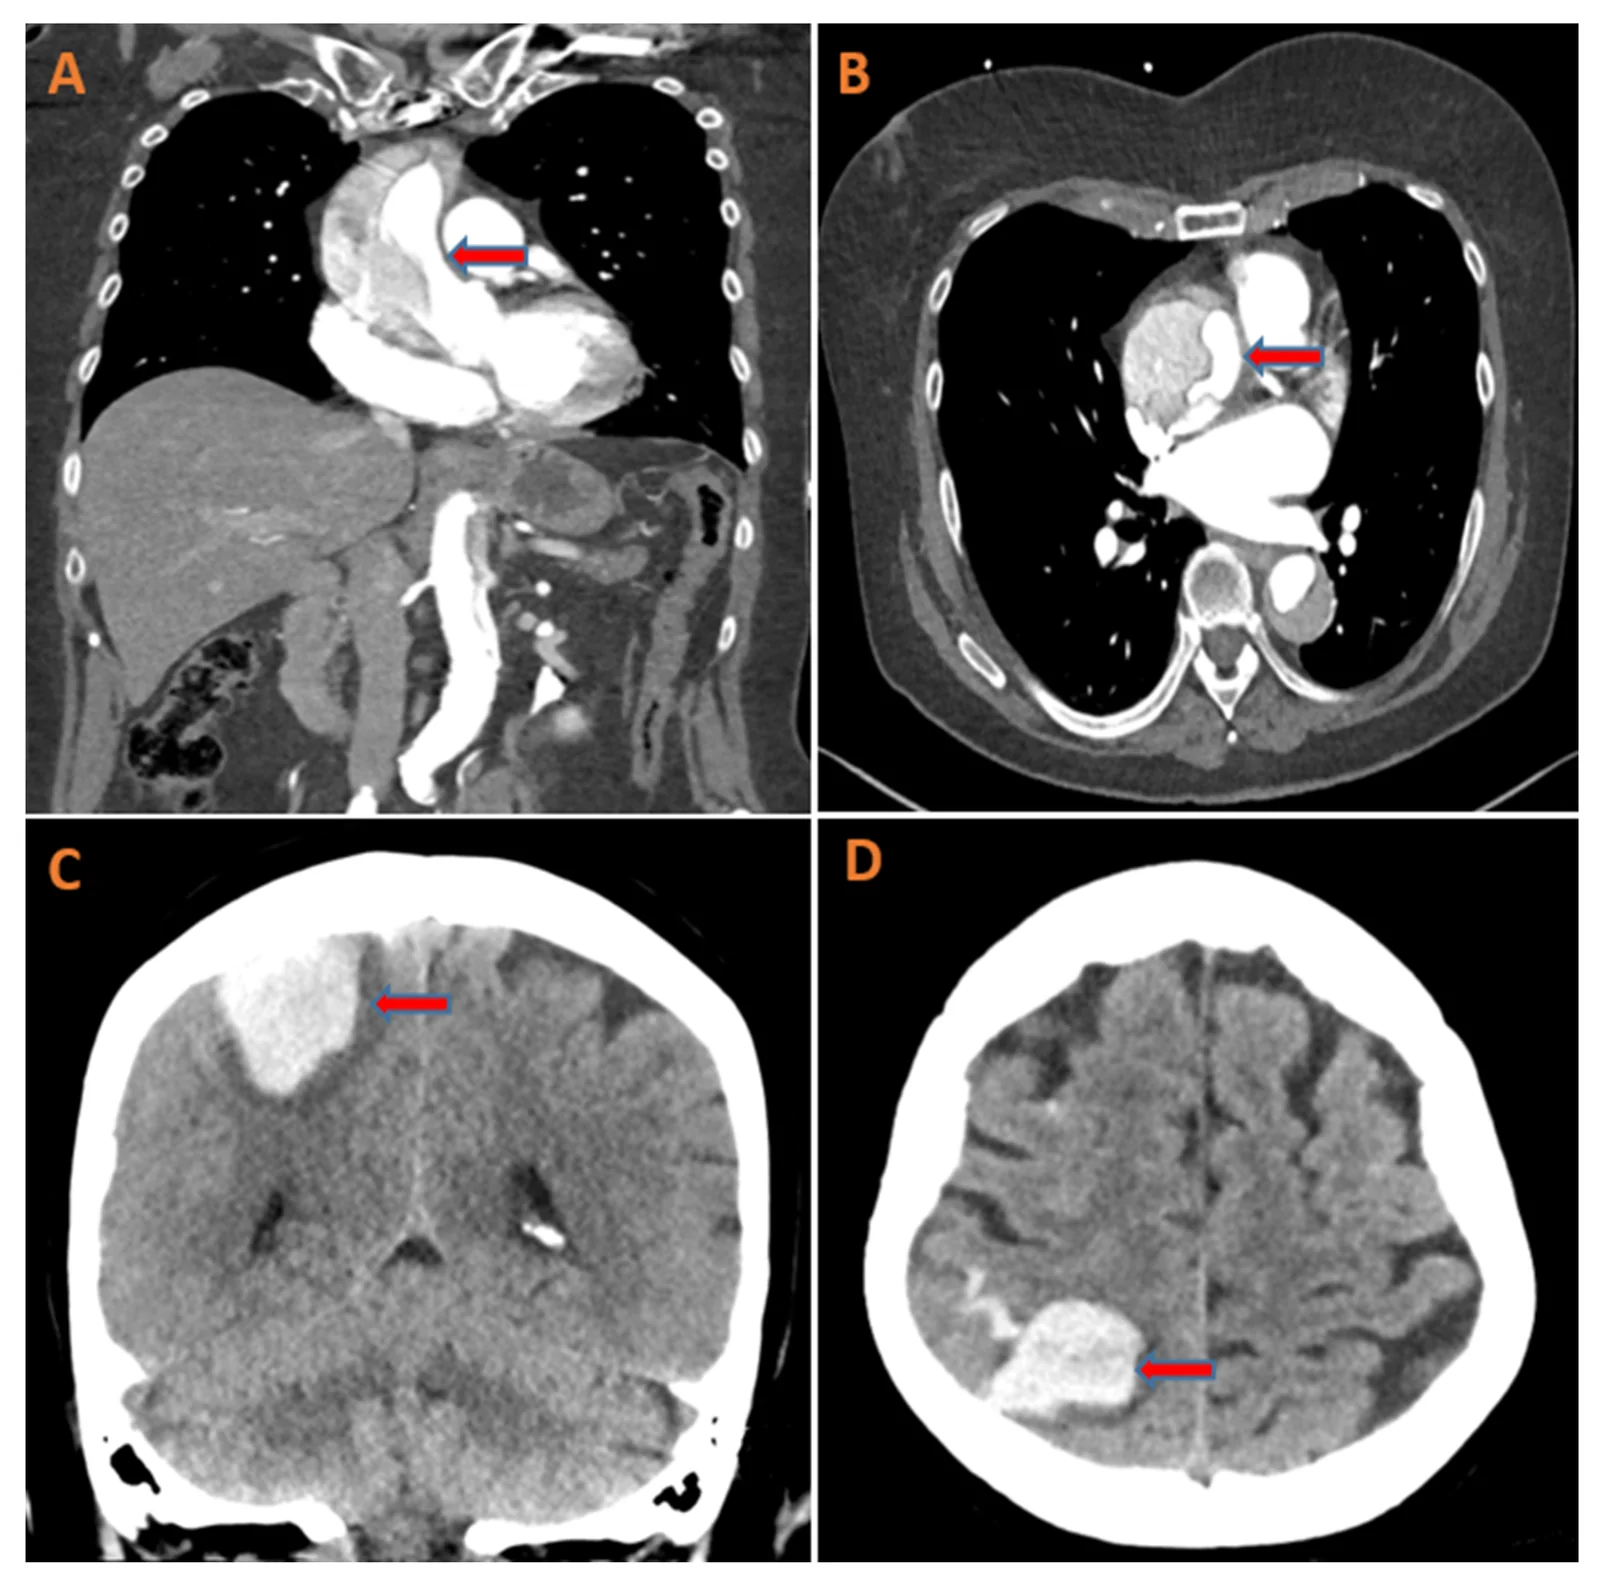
Preoperative computed tomography demonstrating Stanford type A aortic dissection (A,B) and intracerebral haemorrhage extending beyond the high parietal and right frontal regions (C,D).
